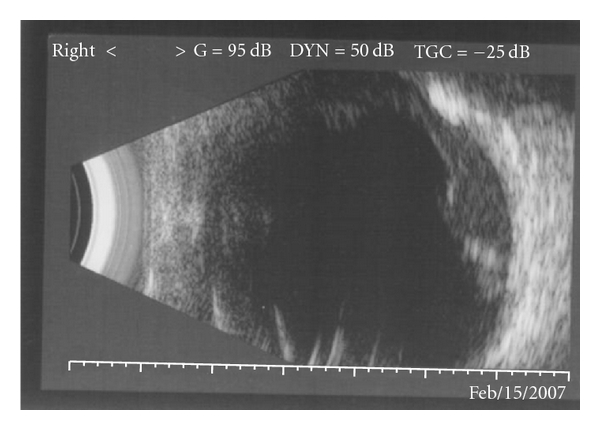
Ultrasound B scan of the left eye showing a subhyaloid haemorrhage and overlying retinal detachment.
